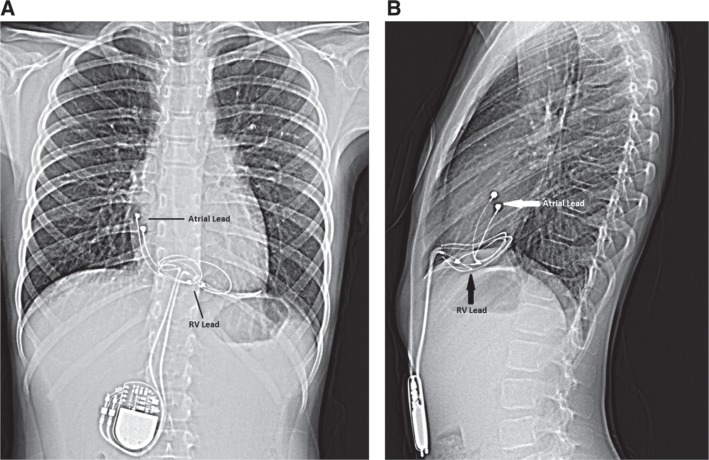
A: Anteroposterior chest radiograph showing lead placement of a bipolar dual-chamber epicardial pacing system in a structurally normal heart. B: Lateral chest radiograph showing lead placement of a bipolar dual-chamber epicardial pacing system in a structurally normal heart.
